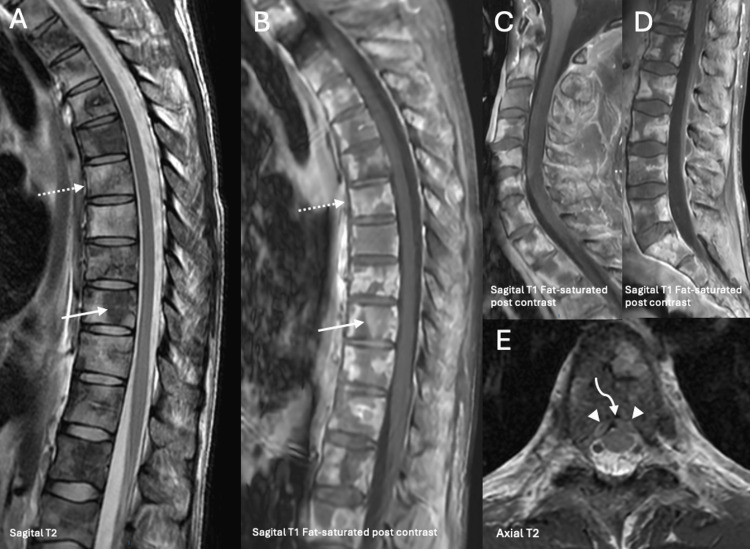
MRI showing heterogeneous signal throughout the cervical (C), thoracic (A, B), and lumbar spine (D) on pre- (A) and post-contrast sequences (B, C, and D), consistent with mixed sclerotic (white arrow) and lytic (dashed arrow) osseous metastatic disease. At the T6 level (E), anterior epidural metastatic extension demonstrates the “draped curtain sign” (white arrows), whereby posterior extension of a vertebral body lesion displaces the posterior longitudinal ligament. The characteristic contour reflects relative preservation of the medial meningovertebral ligament (curved arrow), whose firm midline attachment tethers the lesion centrally, resulting in a bilobulated intracanalicular appearance on axial images.
